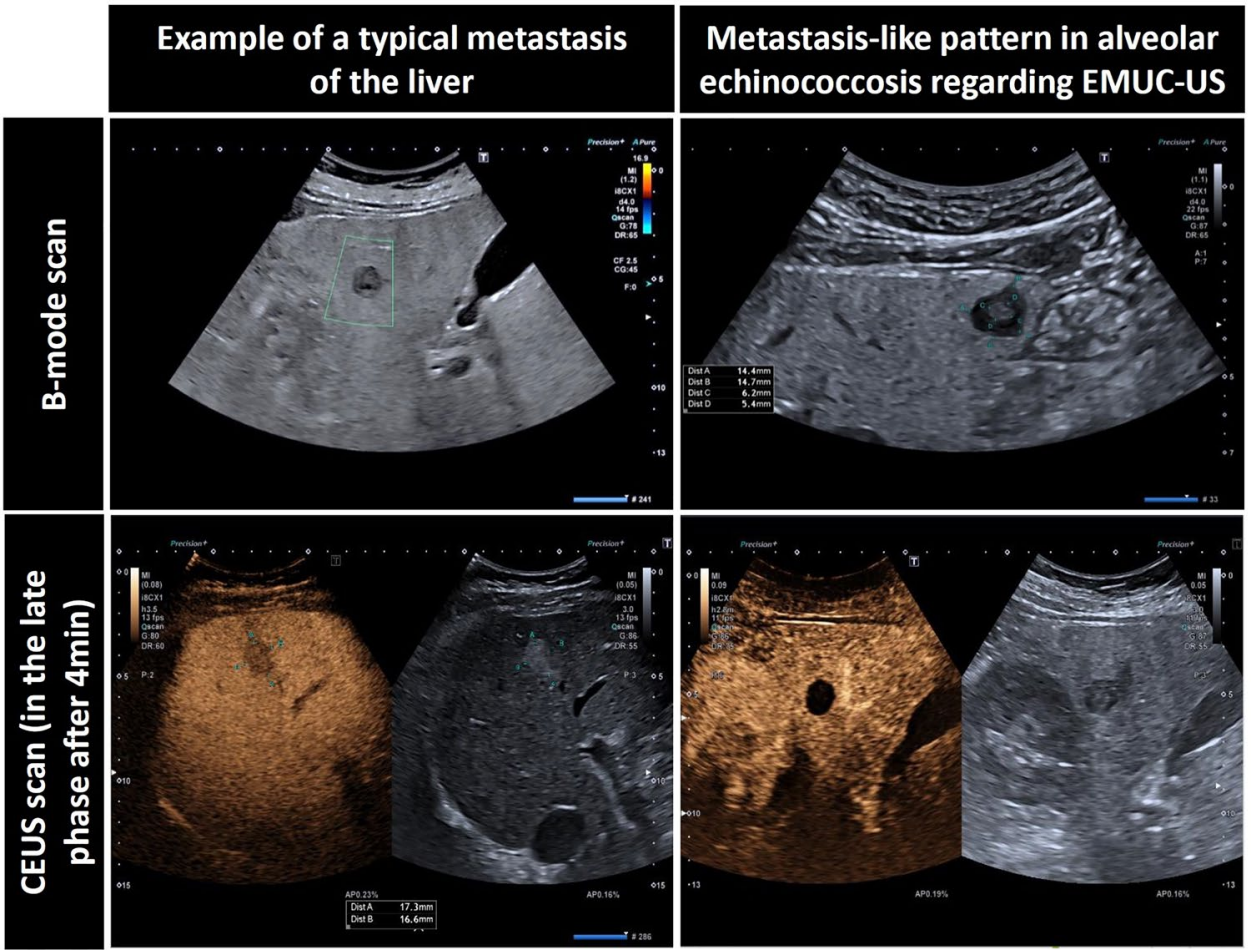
The figure shows a typical liver metastasis as well as a metastasis-like pattern in alveolar echinococcosis according to EMUC-US in the B-scan as well as the corresponding contrast behavior in the late phase after 4 min in contrast-enhanced ultrasound (CEUS). Source: Kratzer W, Weimer H, Schmidberger. Echinokokkose: eine Herausforderung der Lebersonographie. Ultraschall in Med 2021. https://doi.org/10.1055/a-1694-5552
